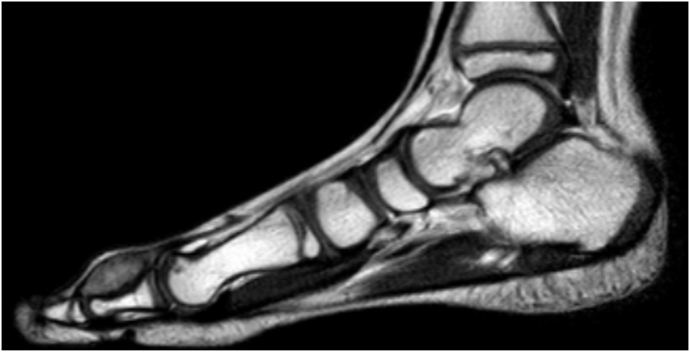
MRI of the foot (Sagital T2WI) shows a well defined fusiform shape soft tissue mass on the dorsum of proximal phalanx of the big toe, with intermediate signal intensity, scalloping underlying bone without destruction.
